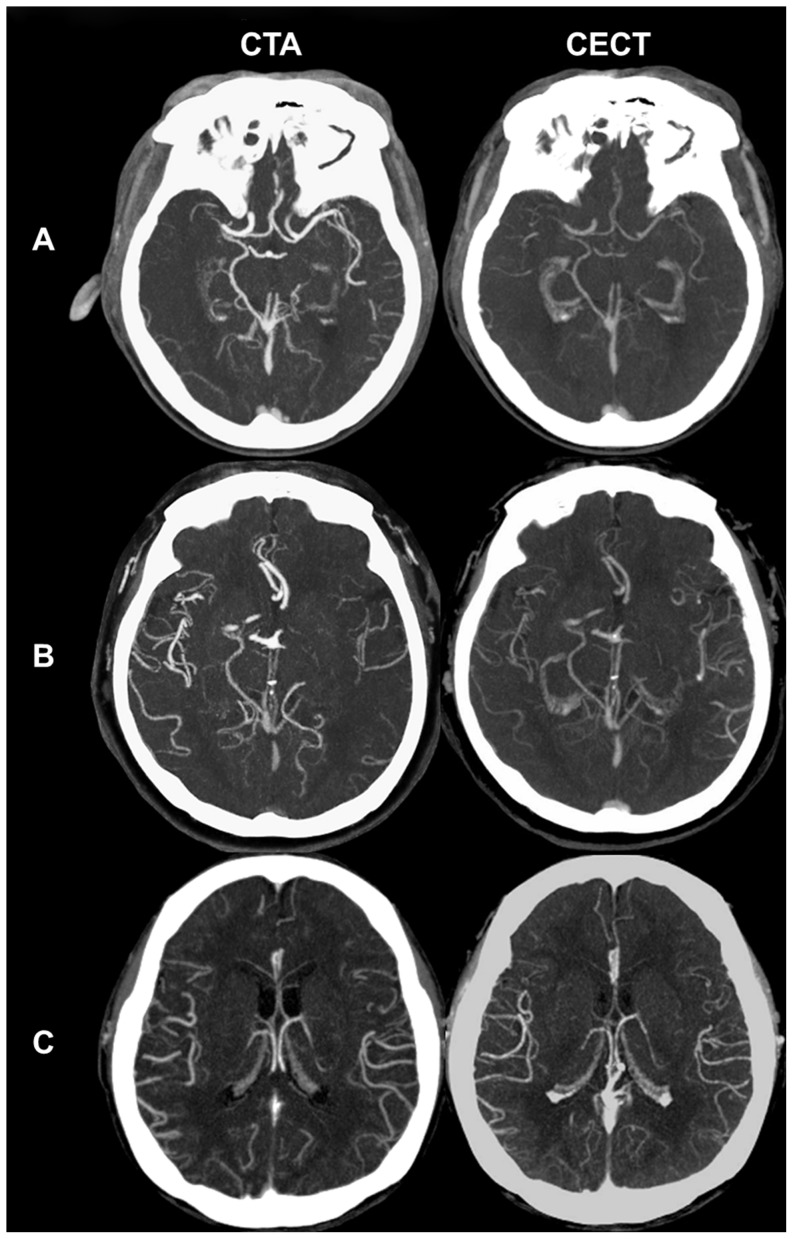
Representative images of collateral status on dual-phase CT. A, Some collaterals to the right MCA territory on CTA with persistence of some defects on CECT (Grade 1). B, Collaterals in part of the left MCA territory on CTA with complete filling on CECT (Grade 2). The collaterals show lower attenuation on CTA with equal to higher attenuation on CECT than unaffected vessels, suggesting slow inflow and washout of collaterals. C, Collaterals in the entire right MCA territory on both CTA and delayed CECT (Grade 3). The collateral vessels show attenuation similar to unaffected vessels on both phase images, suggesting fast velocity of collaterals.
